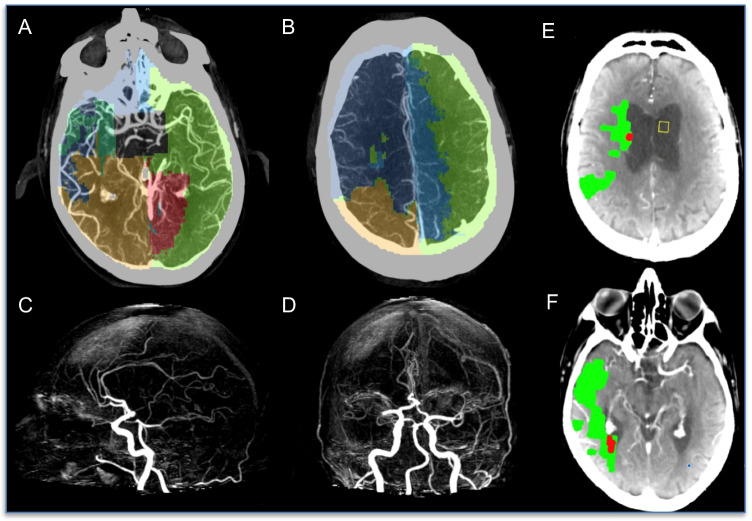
Right MCA occlusion with excellent collaterals. Occluded right M1 segment occlusion. A, B Axial slices of CTA with VTM overlay. C, D CTA MIP demonstrates occlusion of the right MCA at the horizontal segment. E, F MIStar CT Perfusion summary core(red)/penumbra(green) maps (red: CBF < 30% volume, green: DT > 3 volume). VTM software-derived vascular regions show the region supplied by the right MCA is very small (dark green on lower slice A and none on upper slice, B) with a compensatory equal increase of both ACA and PCA regions on the ipsilateral side. The left hemisphere retains the normal VTM pattern. The penumbral region (green) is identified on CT perfusion extending from the right internal capsule to the temporoparietal region. There is minimal perfusion core due to excellent collateralization (demonstrated on both VTM and CTA). On CTP penumbra volume is 83 ml and core is 6 ml. Notably, since collateral flow is very good, some areas of the ischemic MCA territory (supplied by ACA on VTM) have only small delays in contrast to arrival (i.e. delay time < 3 s) and so are under the penumbral threshold (DT > 3 s)
